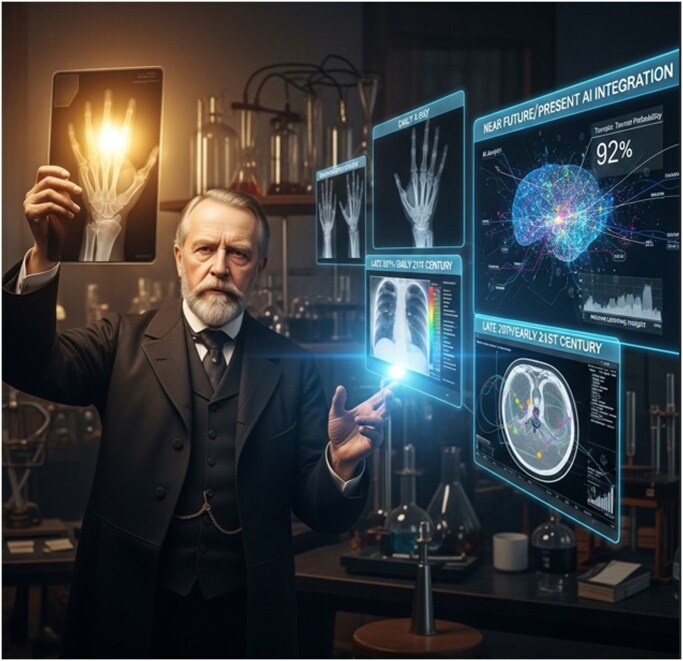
This image represents the transformation of radiology from the invention of X-rays to the present by embracing new technologies.
